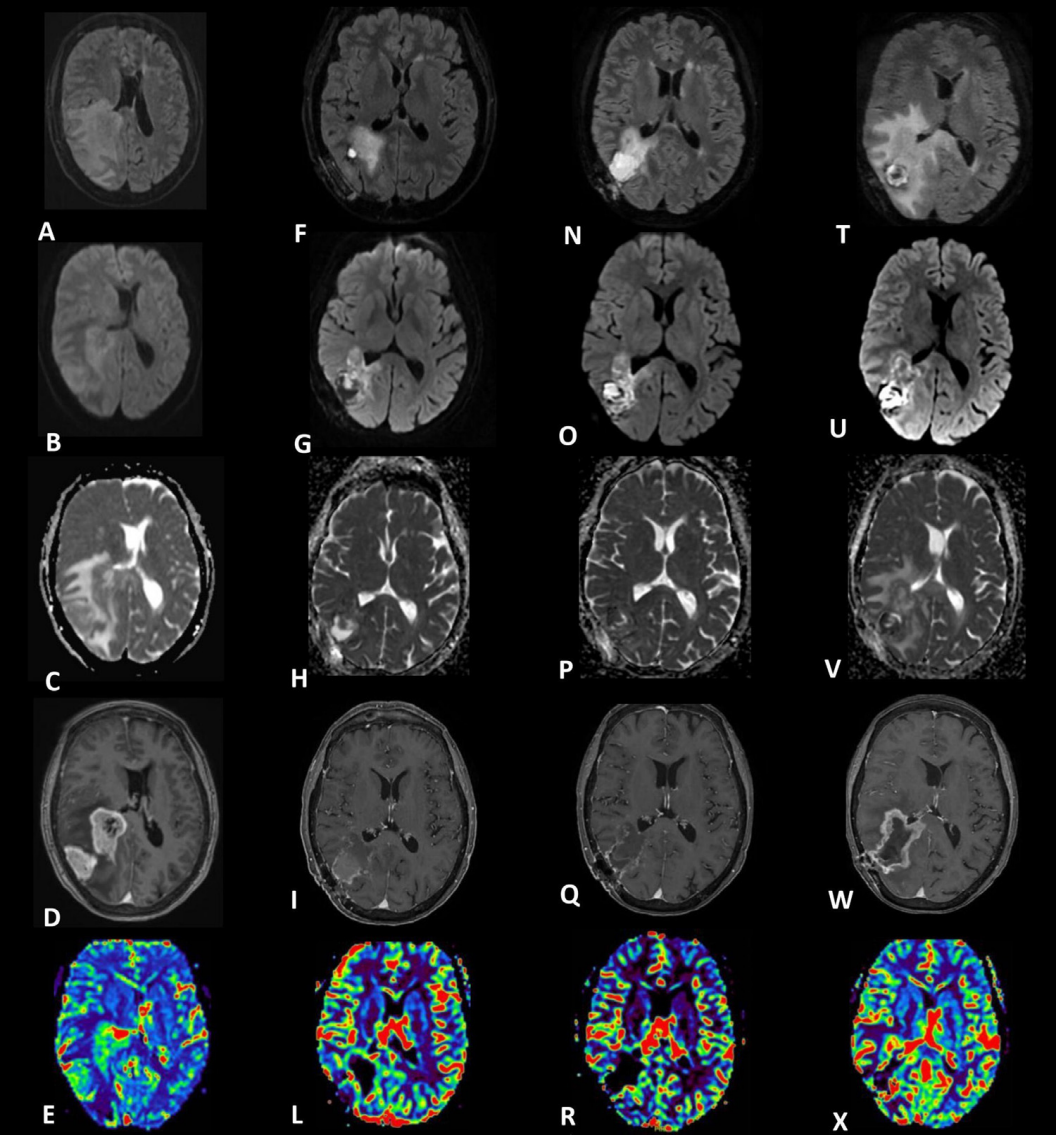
MRI investigations performed at our institution following tumor protocol comprising fluid attenuated inversion recovery (FLAIR) images (first row), diffusion weighted images (second row), apparent diffusion coefficient (third row), 3D fast spoiled gradient echo (FSPGR) post-contrastographic T1-weighted images (fourth row), dynamic susceptibility contrast perfusion images (fifth row). Initial magnetic resonance imaging on admission (A-E) 2 lesions in the right peritrigonal and temporo-parietal regions characterized by central necrotic areas, restricted diffusion (B and C), and post-contrastographic enhancement (D). Increased intra-lesional rCBV at DSC perfusion due to neoangiogenesis (E). Extensive perilesional edema with compressive effects on the right lateral ventricle and a 13 mm leftward midline shift (A). Brain MR scans after corticosteroid therapy (F-X). A follow-up scan at three weeks post biopsy and initiation of corticosteroid therapy (F-L) revealed a notable decrease in mass effect and contrast enhancement in the surgically untouched pathological tissue of the right peritrigonal region. Elevated perfusion parameters still present (L), with marked reduction of the vasogenic edema and consequently of the compressive effect on the ventricles, leading to a re-expansion of the ventricular trigone and a realignment of the midline (F). Follow-up MR 5 weeks after initiating corticosteroid treatment (N-R) showed a slight further decrease in the post-contrastographic enhancement at the periphery of the remaining pathological tissue (Q), with the other radiological findings remaining stable. Follow-up MR 9 weeks after tapering DEX down to its minimum dosage (2mg a day) and starting adjuvant radio-chemotherapy (T-X) showed tumor recurrence, evident by an increase in both size and extent of post-contrast enhancement (W). Increased perilesional edema (T) with a 5 mm left midline shift. Pathological increase in intralesional rCBV at DSC perfusion (X).
